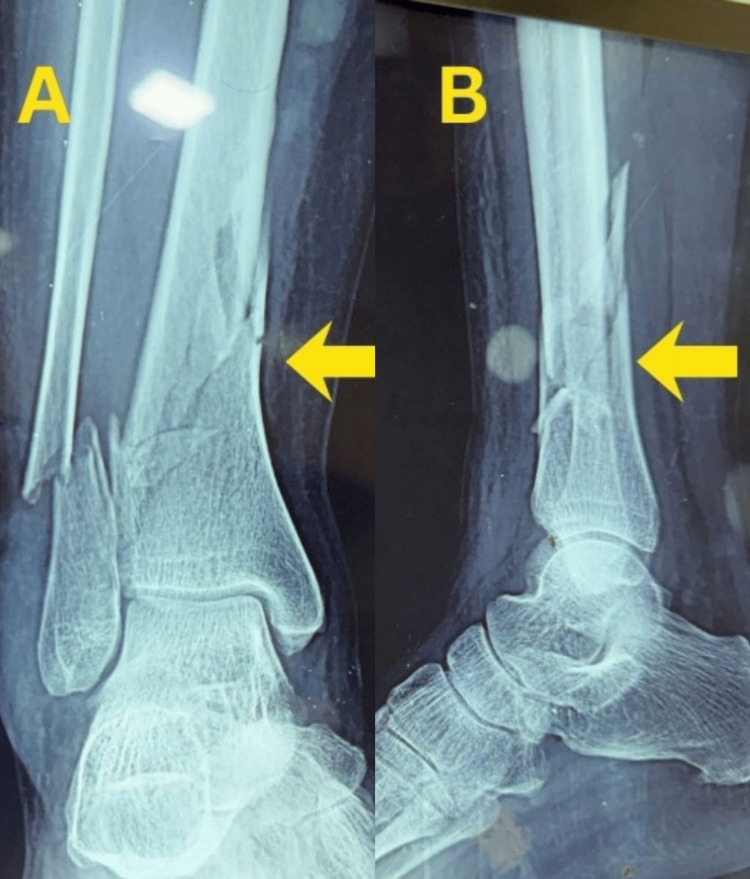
Preoperative radiographs of Case 2. (A) Anteroposterior view showing an extra-articular distal tibial fracture with an associated fibular fracture. (B) Lateral view demonstrating short oblique fracture morphology and displacement.
